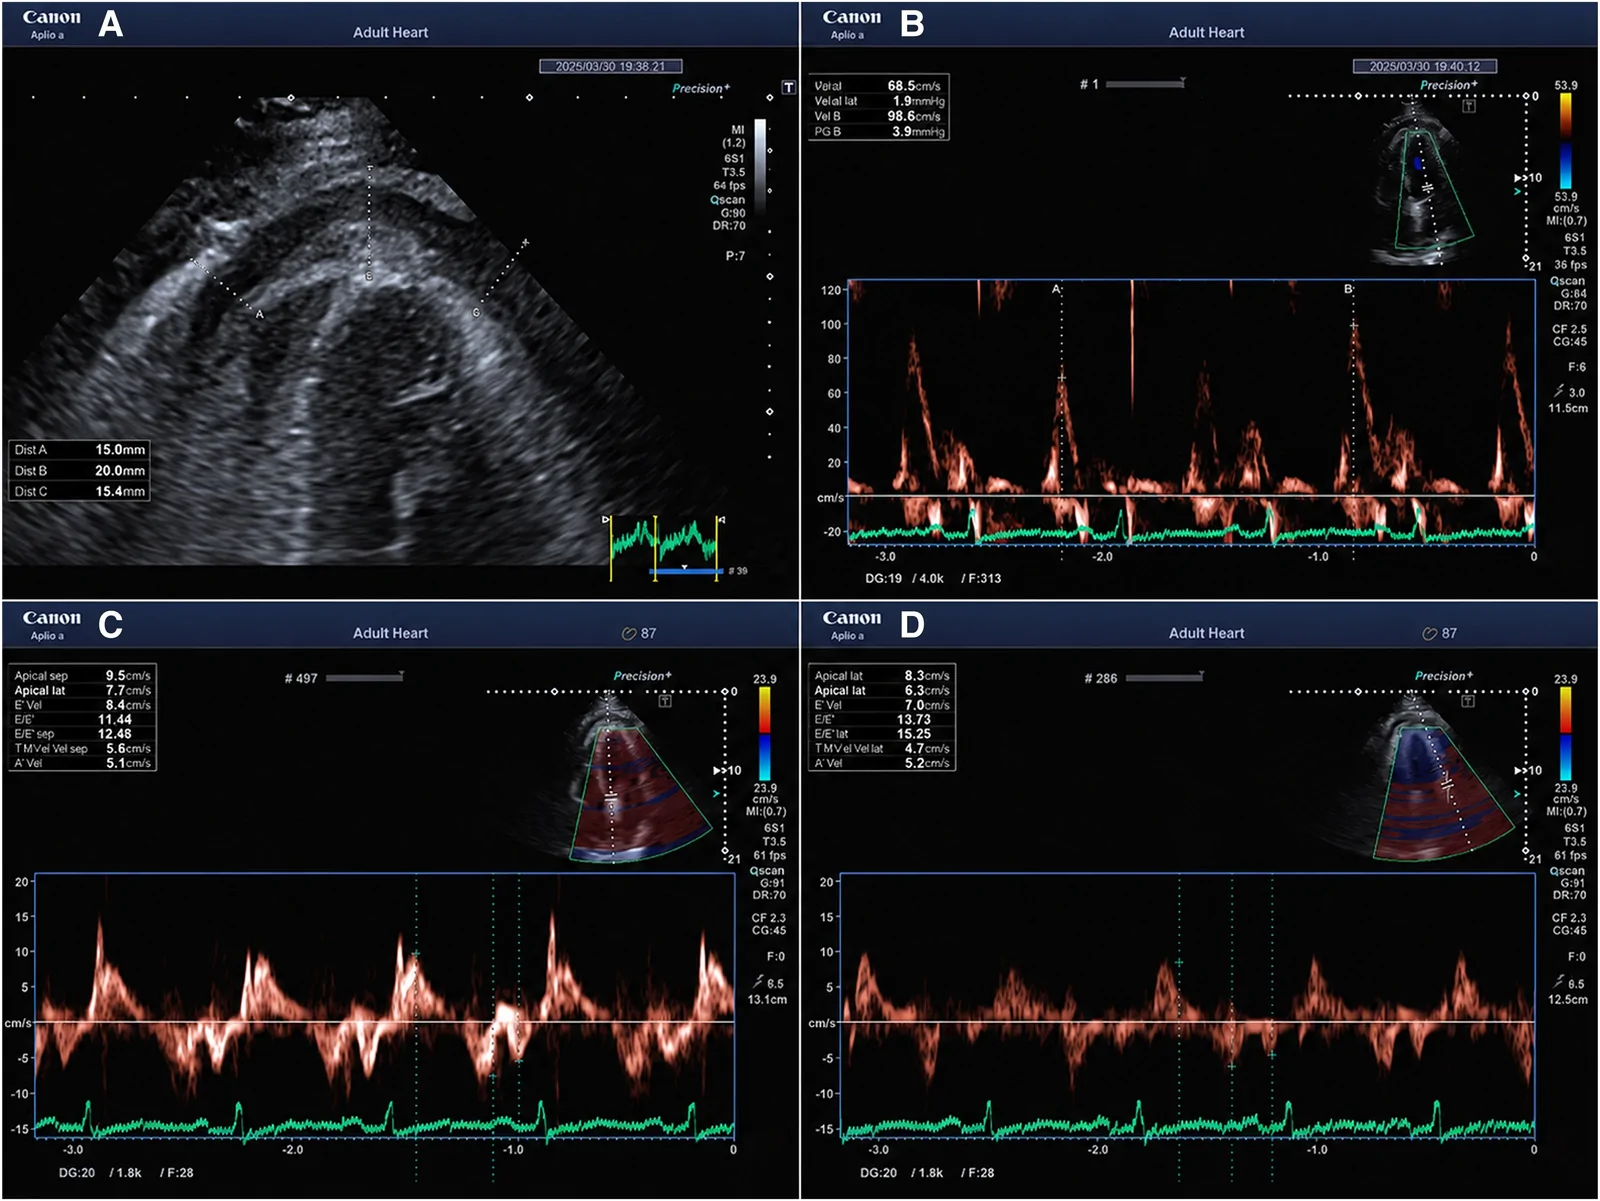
Echocardiographic findings. Transthoracic echocardiography demonstrated marked pericardial thickening of up to 20 mm (*A*), a transmitral E/A ratio > 2 with ∼30% respiratory variation in E-wave velocity (*B*), and markedly reduced lateral mitral annular E′ velocity, consistent with annulus reversus (*C* and *D*).
